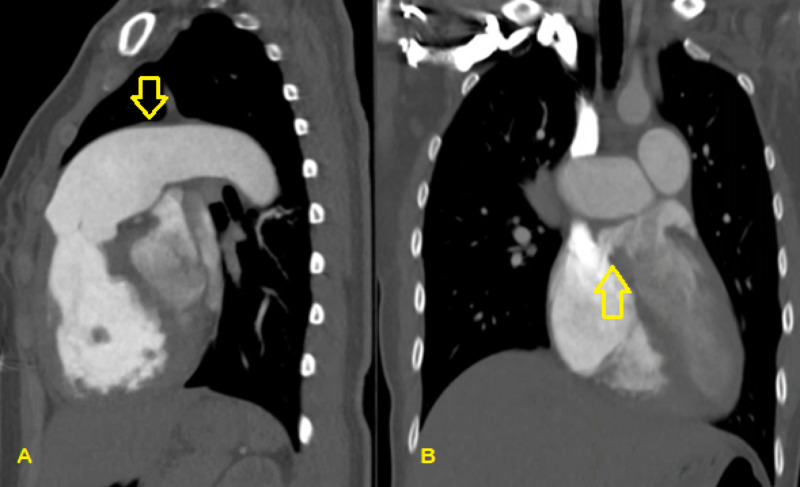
Sagittal (A) and coronal (B) views of a CT chest with contrast showing dilated pulmonary vasculature and atrial septal defect (A) Down arrow indicating 4.4 cm dilation of the main pulmonary artery; (B) up arrow indicating the location of the atrial septal defect
